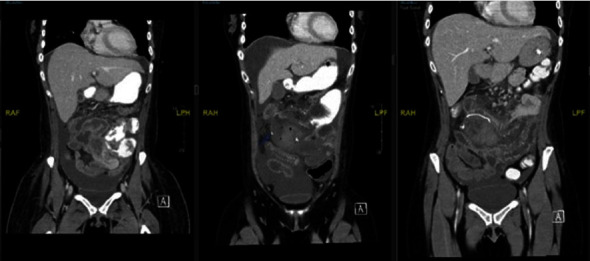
Necrosis of the small bowel. Initially reported as typhlitis but later reported as multiple segments of small bowel ischemia, possible anastomotic breakdown, small bowel obstruction, and enterocolitis. The CT scan shows an ischemic jejunum segment and enterocolitis.
